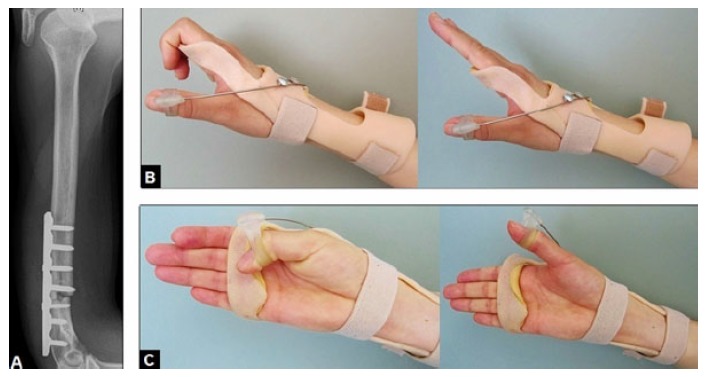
(Principle of our individually customized thermoplastic wrist and hand splint for patients sustaining RNP during both the duration of functional recovery with or without surgery and/or before tendon transfers): (A) Lateral radiograph of a 22-year-old female demonstrating open reduction and internal fixation of a right distal humerus shaft fracture performed in another hospital, there was an iatrogenic RNP postoperatively (axonotmesis); (B) Clinical photographs of the same patient showing that the wrist is immobilized in intrinsic plus position to avoid shortening of the antagonistic extrinsic flexor muscles in order to avoiding a secondary wrist flexion contracture, the MP II-V joints are immobilized in neutral position to avoid shortening of the antagonistic extrinsic flexor muscles of the long fingers in order to avoiding MP joint flexion contractures, the proximal interphalangeal joints are not immobilized because the intrinsic-related extension in these joints is not affected by RNP; (C) Clinical photographs of the same patient showing that the non-affected extrinsic-related flexion of the thumb is completely freed whereas the affected extrinsic-related extension and abduction of the thumb is passive realized by a flexible steel feather which is dorsally placed at the splint combined with alignment of the thumb in an abduction position, a spontaneous complete functional recovery was observed 7 months postoperatively.
